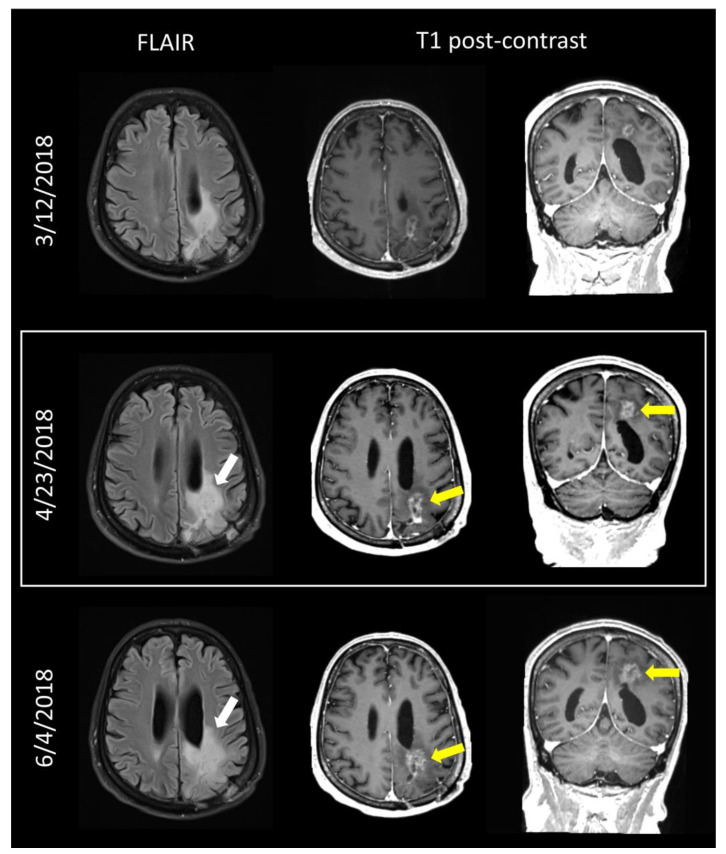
Images from a 71 year-old man with IDH wild-type glioblastoma. Images from 4/23/2018 (middle row, white box) were reviewed at tumor board. Compared with prior study (top row), both FLAIR (white arrow) and enhancing tumor volume were increasing (yellow arrows). While the radiology report called this study a 3b, the tumor board presenter and the tumor board consensus felt the increase was sufficient to score it as disease progression (BT-RADS 4). Subsequent follow-up (bottom row) showed further increase (arrows) and the patient underwent re-irradiation of recurrent disease.
